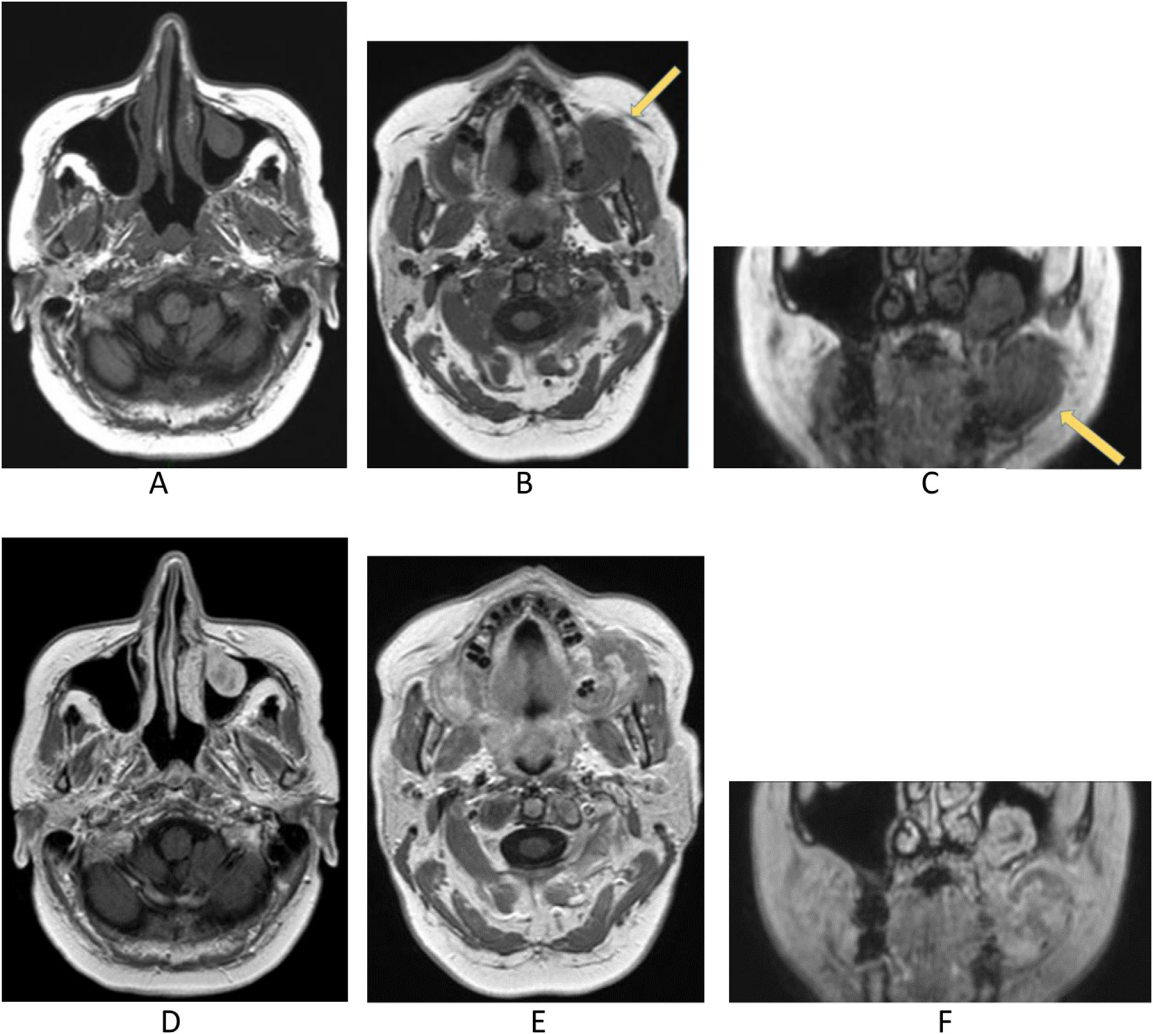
(A-F) MRI T1 SE sequences before contrast medium administration, axial plane (A,B) and coronal plane (C). The lesion showed low-intermediate signal intensity on T1-weighted sequence. The presence of only one lesion extending from the left maxillary sinus to masticator space with erosion of the sinus floor was better evident on the coronal plane (C). After contrast medium administration (D-F) the lesion showed inhomogeneous contrast-enhancement.
